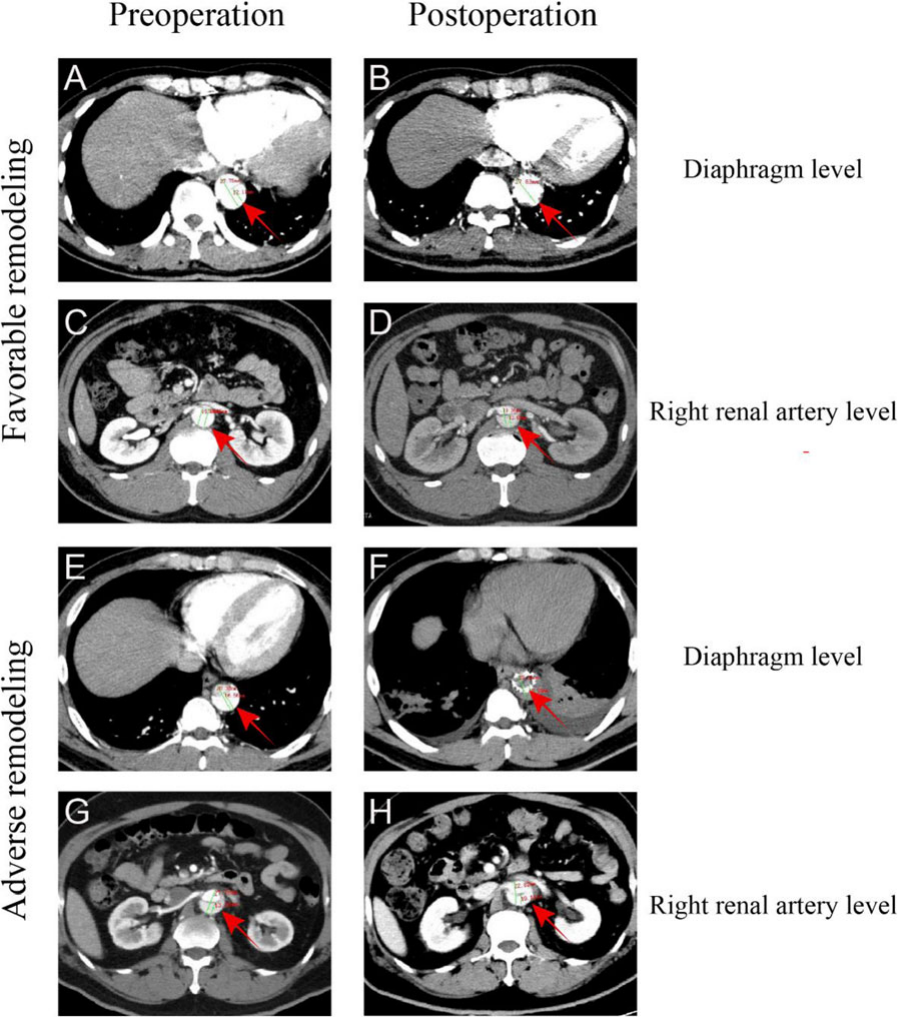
CT images, before surgery and after TEVAR, showing favorable and adverse aortic remodeling. A and B: Favorable aortic remodeling before and after surgery observed at the diaphragm level. The false lumen had almost disappeared. C and D: Favorable aortic remodeling before and after surgery observed at the level of the right renal artery. The false lumen had almost disappeared. E and F: Adverse aortic remodeling before and after surgery observed at the diaphragm level. The false lumen obviously enlarged. G and H: Adverse aortic remodeling before and after surgery observed at the level of the right renal artery. The false lumen had no obviously change after 6 months. Red arrows indicate the location of dissected lesions
